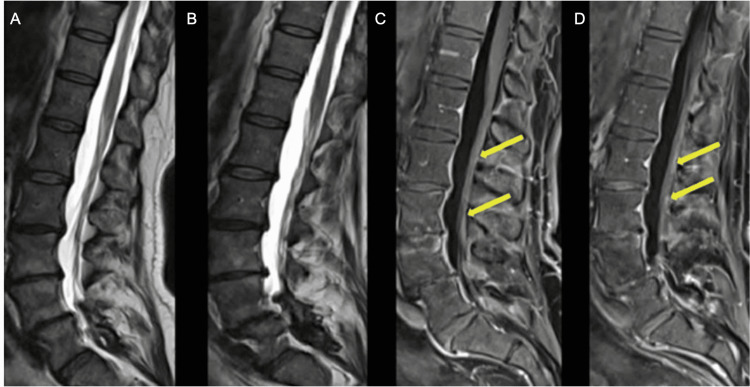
MRI of the lumbar spine with and without contrast A and B: Sagittal T2 sequence demonstrating T2 hyperintensities in cauda equina roots. C and D: Sagittal T1 post-contrast sequence demonstrating Gadolinium enhancement of the cauda equina roots.
